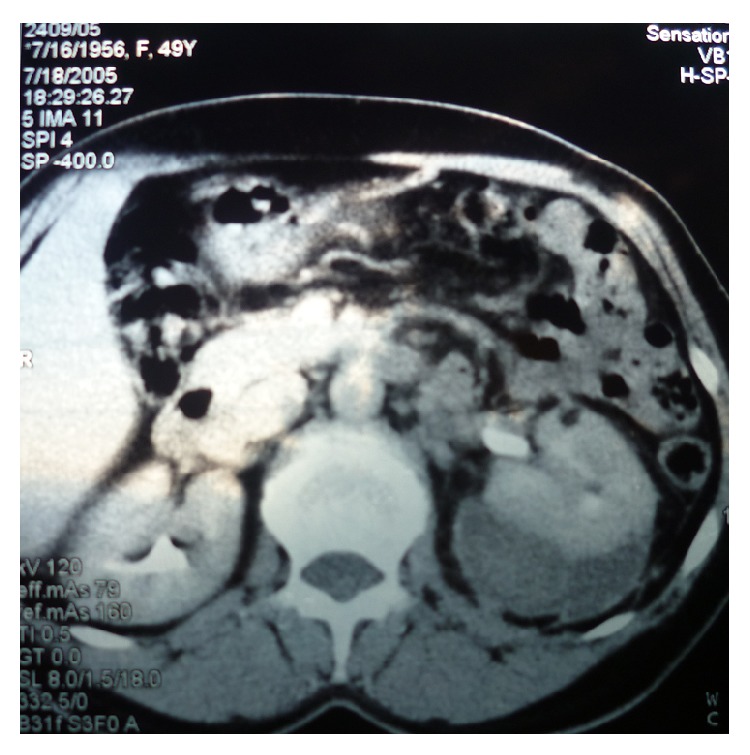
CT scan revealed a perirenal hematoma around the left kidney with an angiomyolipoma in the ventral part of the kidney.
